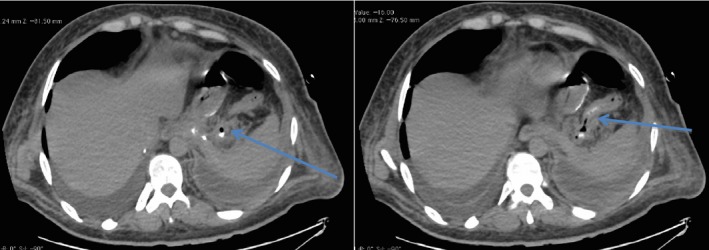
CT abdomen with oral contrast showing leak of oral contrast from the gastric pouch into the distal transverse colon (arrows point to the fistula), note resolution of left pleural effusion.
